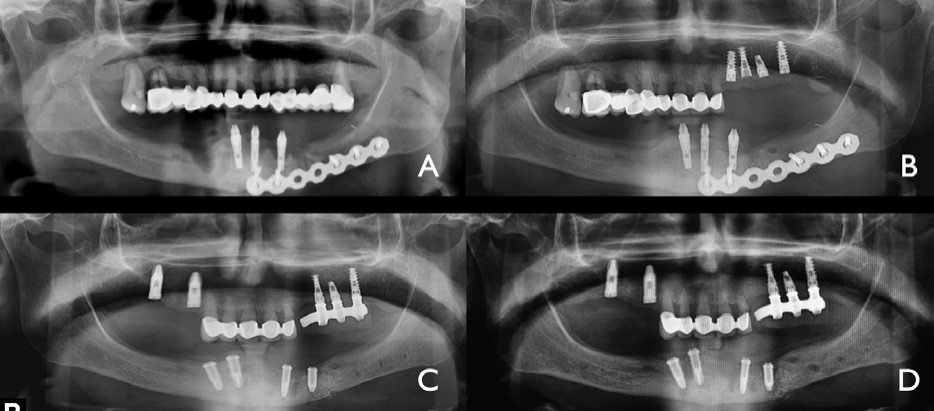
Case 2: A) Panoramic radiography showing rigid fixation using a reconstruction plate. B) Eight months later, a good healing of mandibular fracture is observed. C) The titanum plate is removed. New mandibular and maxillary implants are placed. D) One year later, mild periimplant bone loss is observed around implants.
